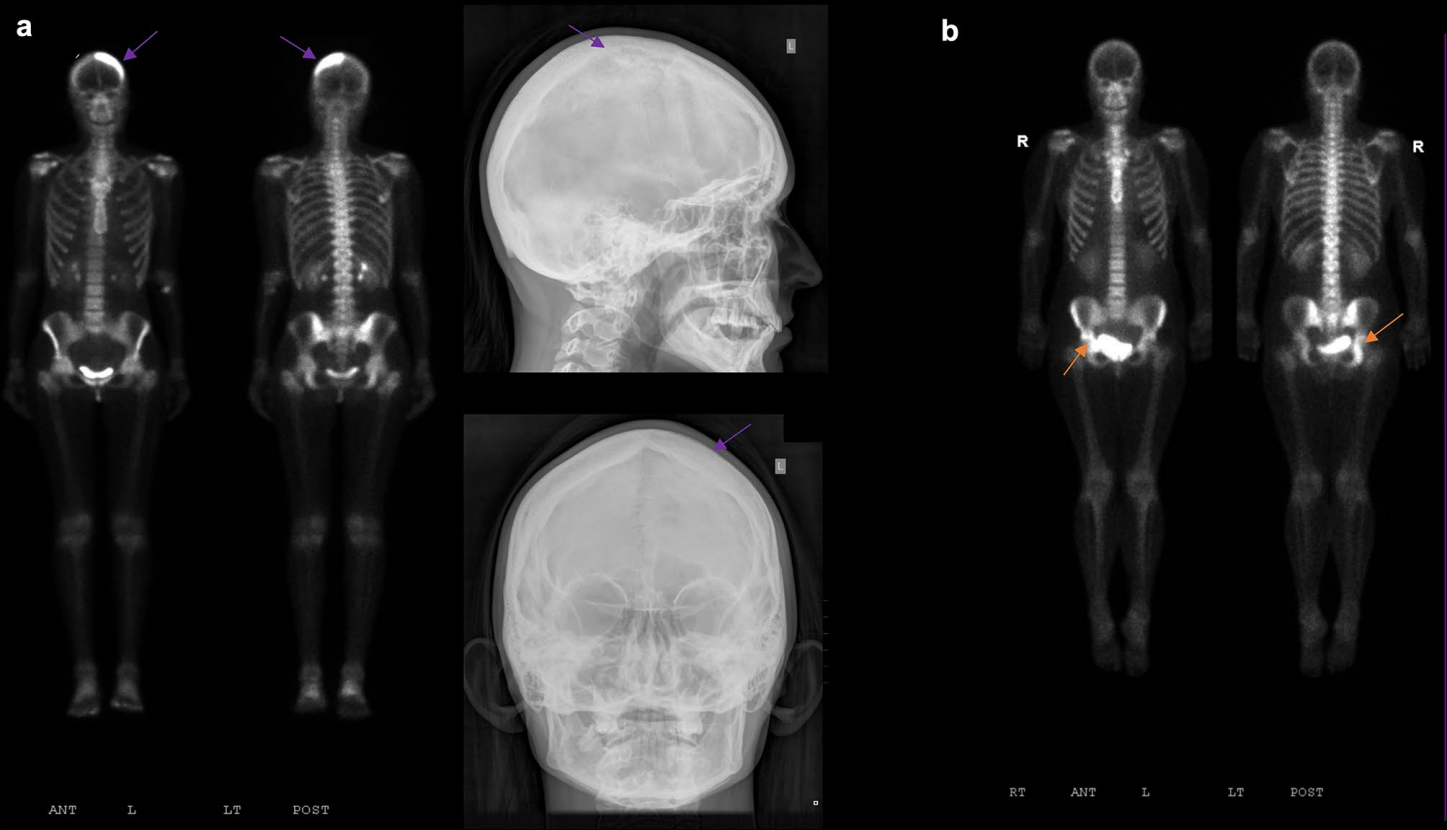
Whole body 99mTc-MDP bone scan and skull radiographs in two newly diagnosed PDB in myopathy only patients. (a) A 28-years-old female (ID 6) with increased uptake in the skull on the bone scan corresponding to a mixed lytic and sclerotic bone lesion in the left parietal calvarium on the AP and lateral views of the plain radiographs (purple arrows). (b) A 38-years-old female (ID 10) with increased uptake in the right ischium and acetabulum on whole-body bone scan (orange arrows).
